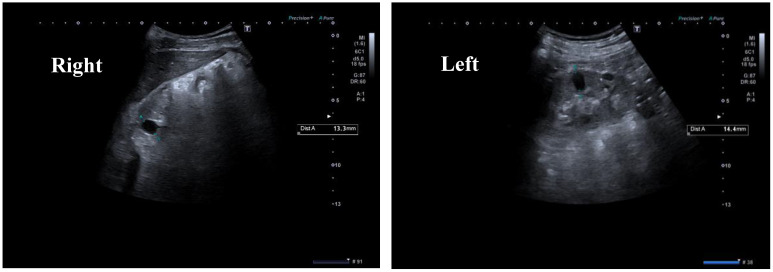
Renal ultrasound revealing bilateral renal cysts.
